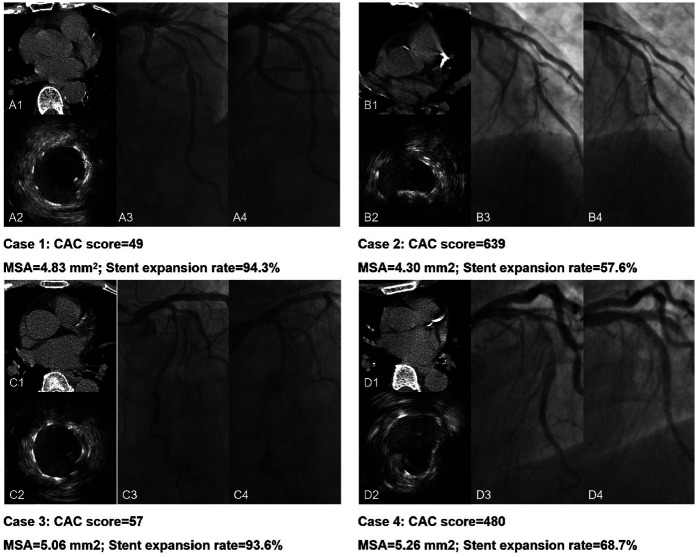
Representative case examples. Case 1. CAC score < 200 in NCCT (A1) and excellent stent expansion (A2); A3 and A4 present the pre- and post-PCI angiography of the LAD lesion. Case 2. CAC score > 200 in NCCT (B1) with stent underexpansion (B2); B3 and B4 present the pre- and post-PCI angiography of the LAD lesion. Case 3. CAC score < 200 in CCTA (C1) and excellent stent expansion (C2); C3 and C4 present the pre- and post-PCI angiography of the LAD lesion. Case 4. CAC score > 200 in CCTA (D1) with stent underexpansion (D2); D3 and D4 present the pre- and post-PCI angiography of the LAD lesion. NCCT, non-gated non-contrast chest computed tomography; CCTA, coronary computed tomographic angiography; PCI, percutaneous coronary intervention; LAD, left anterior descending artery.
